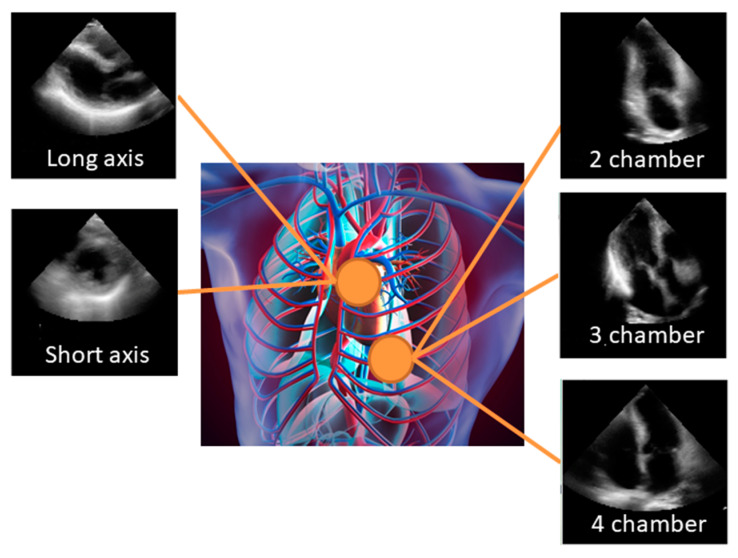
Standard views: The apical 2-chamber (AP2), apical 4-chamber (AP4), apical 3-chamber (AP3), parasternal long axis (PLAX), and parasternal short axis (PSAX) views were stored digitally for playback and analysis. Echocardiographic images shown here are the average of 10 consecutive images.
